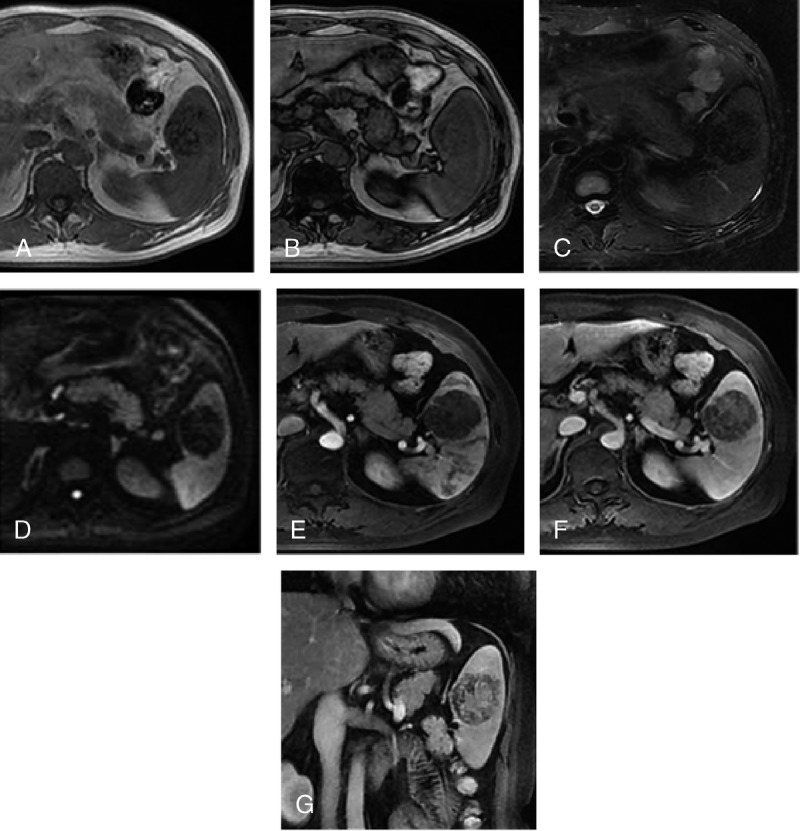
Sclerosing angiomatoid nodular transformation in a 49-year-old man. On the in-phase and out-of-phase images (A, B), the lesion presents heterogeneous hypointensity with ill-defined boundary. On axial T2-weighted and DWIs (C, D), the lesion shows remarkable hyperintense with clear boundary and lobulation. On the contrast-enhanced MR images including arterial (E), portal venous (F), and delayed phase (G), the lesion shows mild heterogeneous enhancement with the pattern of progressive and centripetal, and the typical sign of spoke-wheel is not found.
